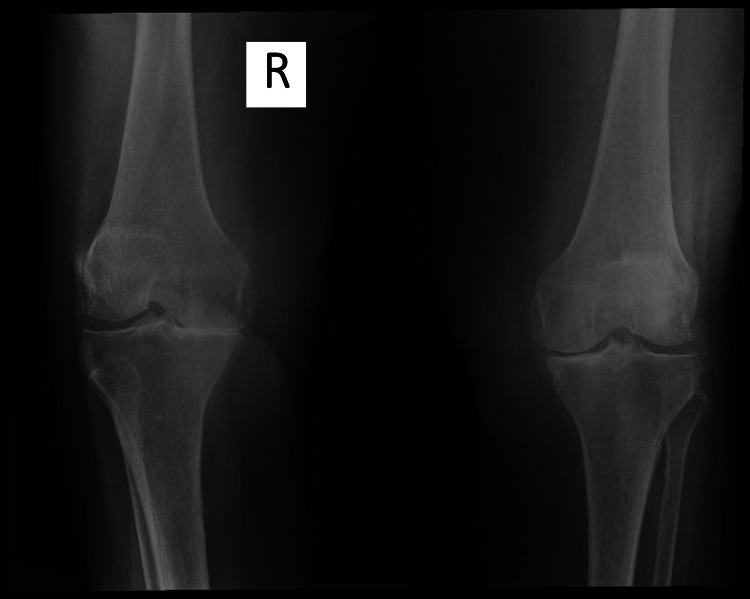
Bilateral knee radiograph showing joint space narrowing in both knees, more accentuated in the medial aspect, and subchondral sclerosis, suggesting gonarthrosis. R: right.
